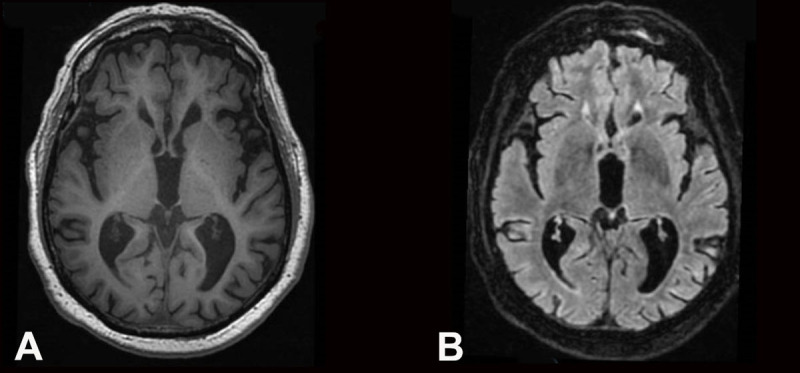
Brain magnetic resonance imaging (MRI) of the patient. A: T1-weighted axial image, and B: fluid attenuated inversion recovery (FLAIR) axial image, showing normal findings in the basal ganglia and Fazekas 1 leucopathy.
